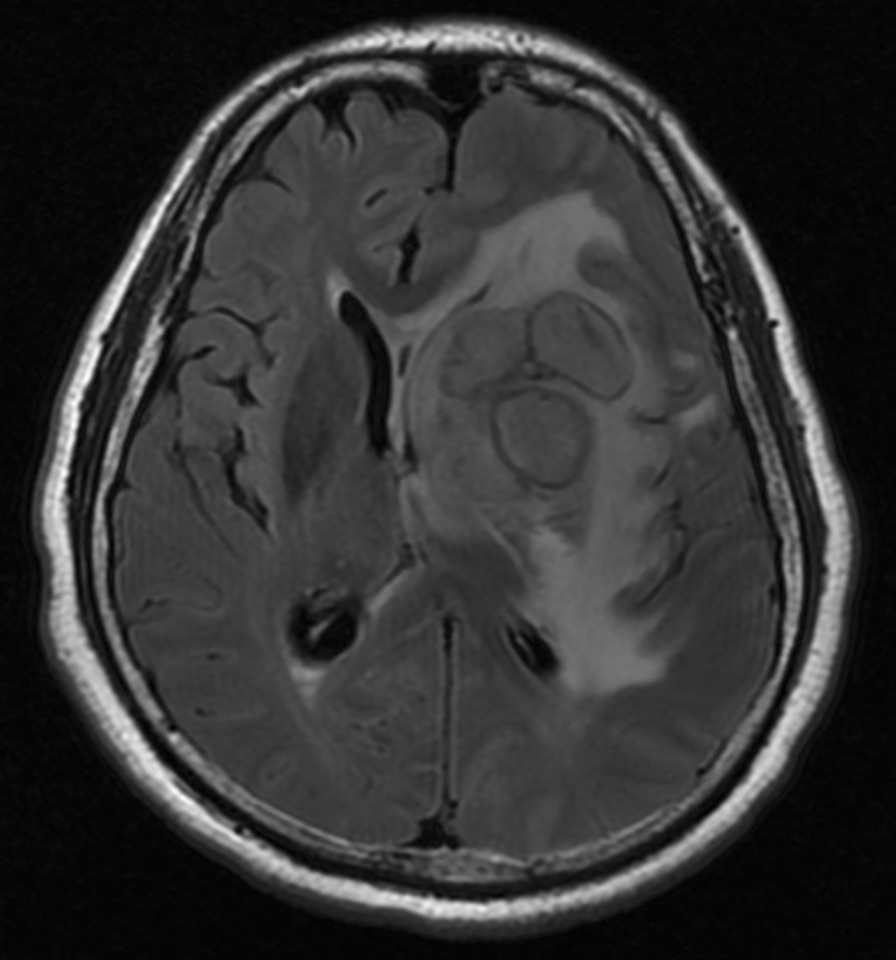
Magnetic resonance imaging of the brain showing a T1 hyperintense, T2 hyperintense heterogeneous lesion with loss of signal on fat-saturated T1 sequence involving right Meckel cave and posterior cerebellopontine angle measuring approximately 3.3 × 1.8 × 1.4 cm
